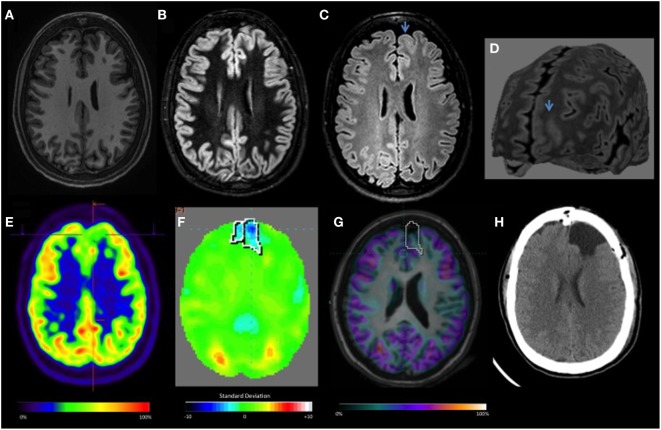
Focal cortical dysplasia II-B left frontal lobe: (A–D) T1, double inversion recovery, FLAIR axial MRI, and curvilinear reconstruction, showing abnormal cortical thickening, focal increased signal, white and gray matter junction blurred (arrow). (E) Visual-positron emission tomography (PET) initially was considered normal. (F) Quantitative-PET showed −3.6 SD in the left frontal lobe. (G) Co-registration MRI and PET. (H) Axial CT after surgery resection.
